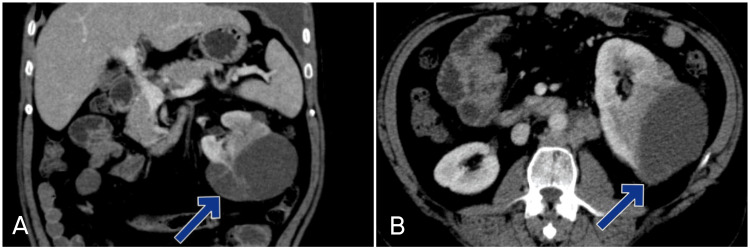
CT scan of the abdomen and pelvis suggestive of a large lobulated solid cystic mass (blue arrows) along the posterior aspect of the left kidney, indicative of malignancy. (A) Coronal section, (B) Axial section.
